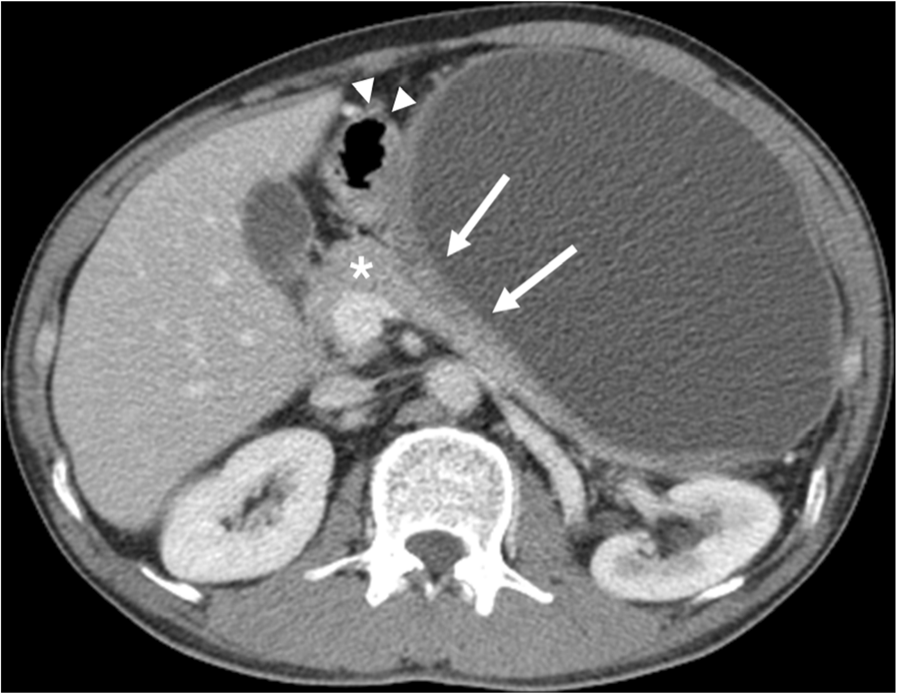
Retroperitoneal desmoid-type fibromatosis in a 46-year-old man. Axial image of CT shows an 18.3 × 12.3 × 21.5 cm sized oval cystic mass with a thick wall (arrows) that has a mass effect on the stomach (arrowheads) and pancreas (asterisk). There is no evidence of an enhancing septum or a solid portion within the cystic mass
